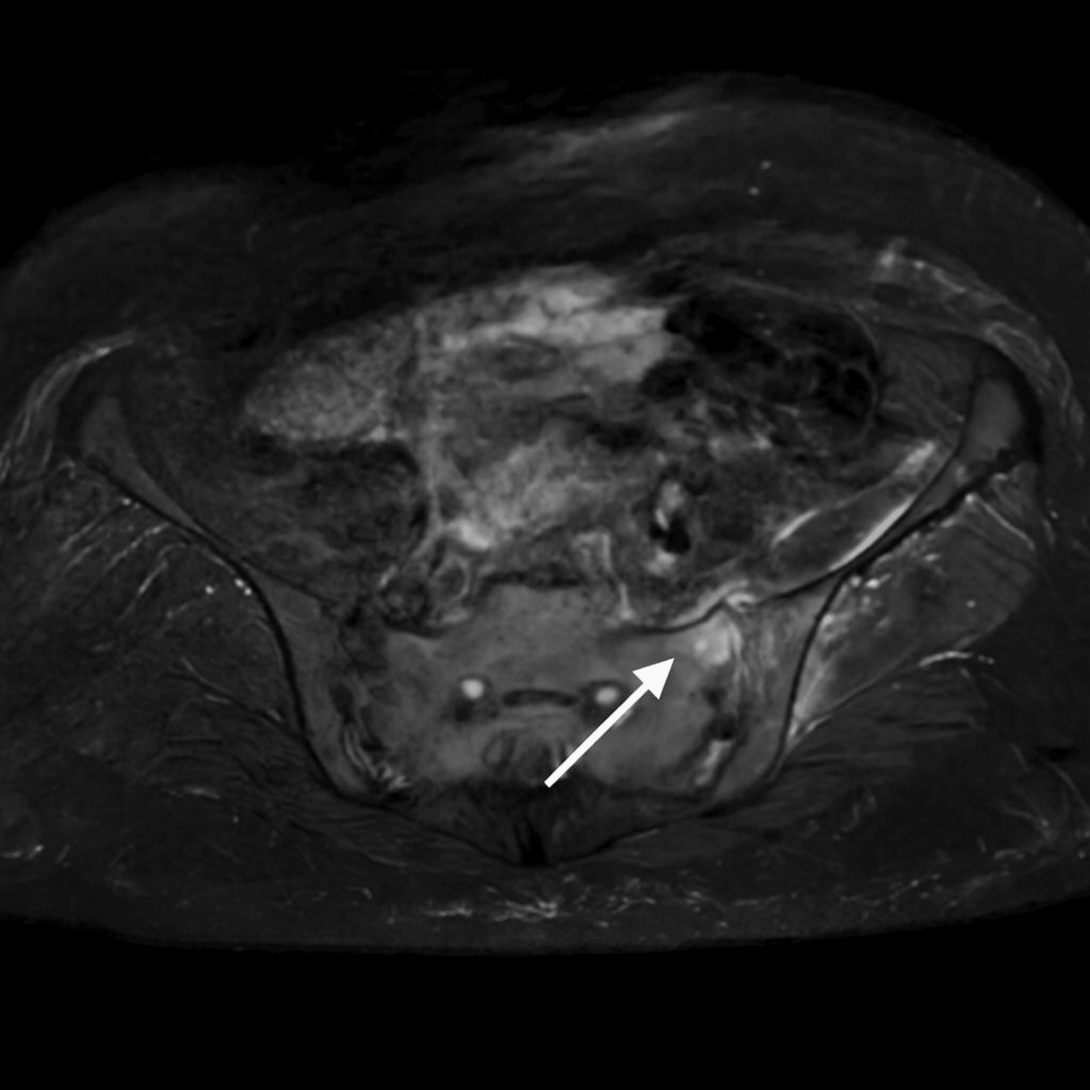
Sagittal T2-weighted MRI. Increased STIR signal in the subchondral bone of the left sacroiliac joint (arrow), confirming sacroiliitis.
